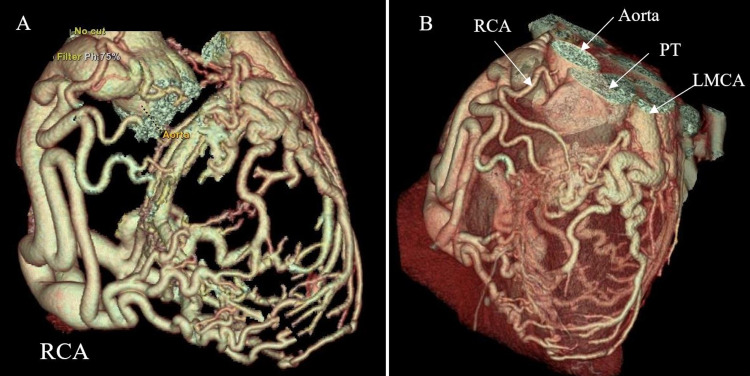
3D volume-rendered CCTA. (A) Dilated and tortuous RCA with multiple collaterals extending to the left ventricle. (B) While the RCA is clearly arising from the aorta, LMCA is arising from the PT. Also, the LMCA is observed in smaller size with very limited extension comparing to the RCA. CCTA, coronary computed tomography angiogram; LMCA, left main coronary artery; PT, pulmonary trunk; RCA, right coronary artery
